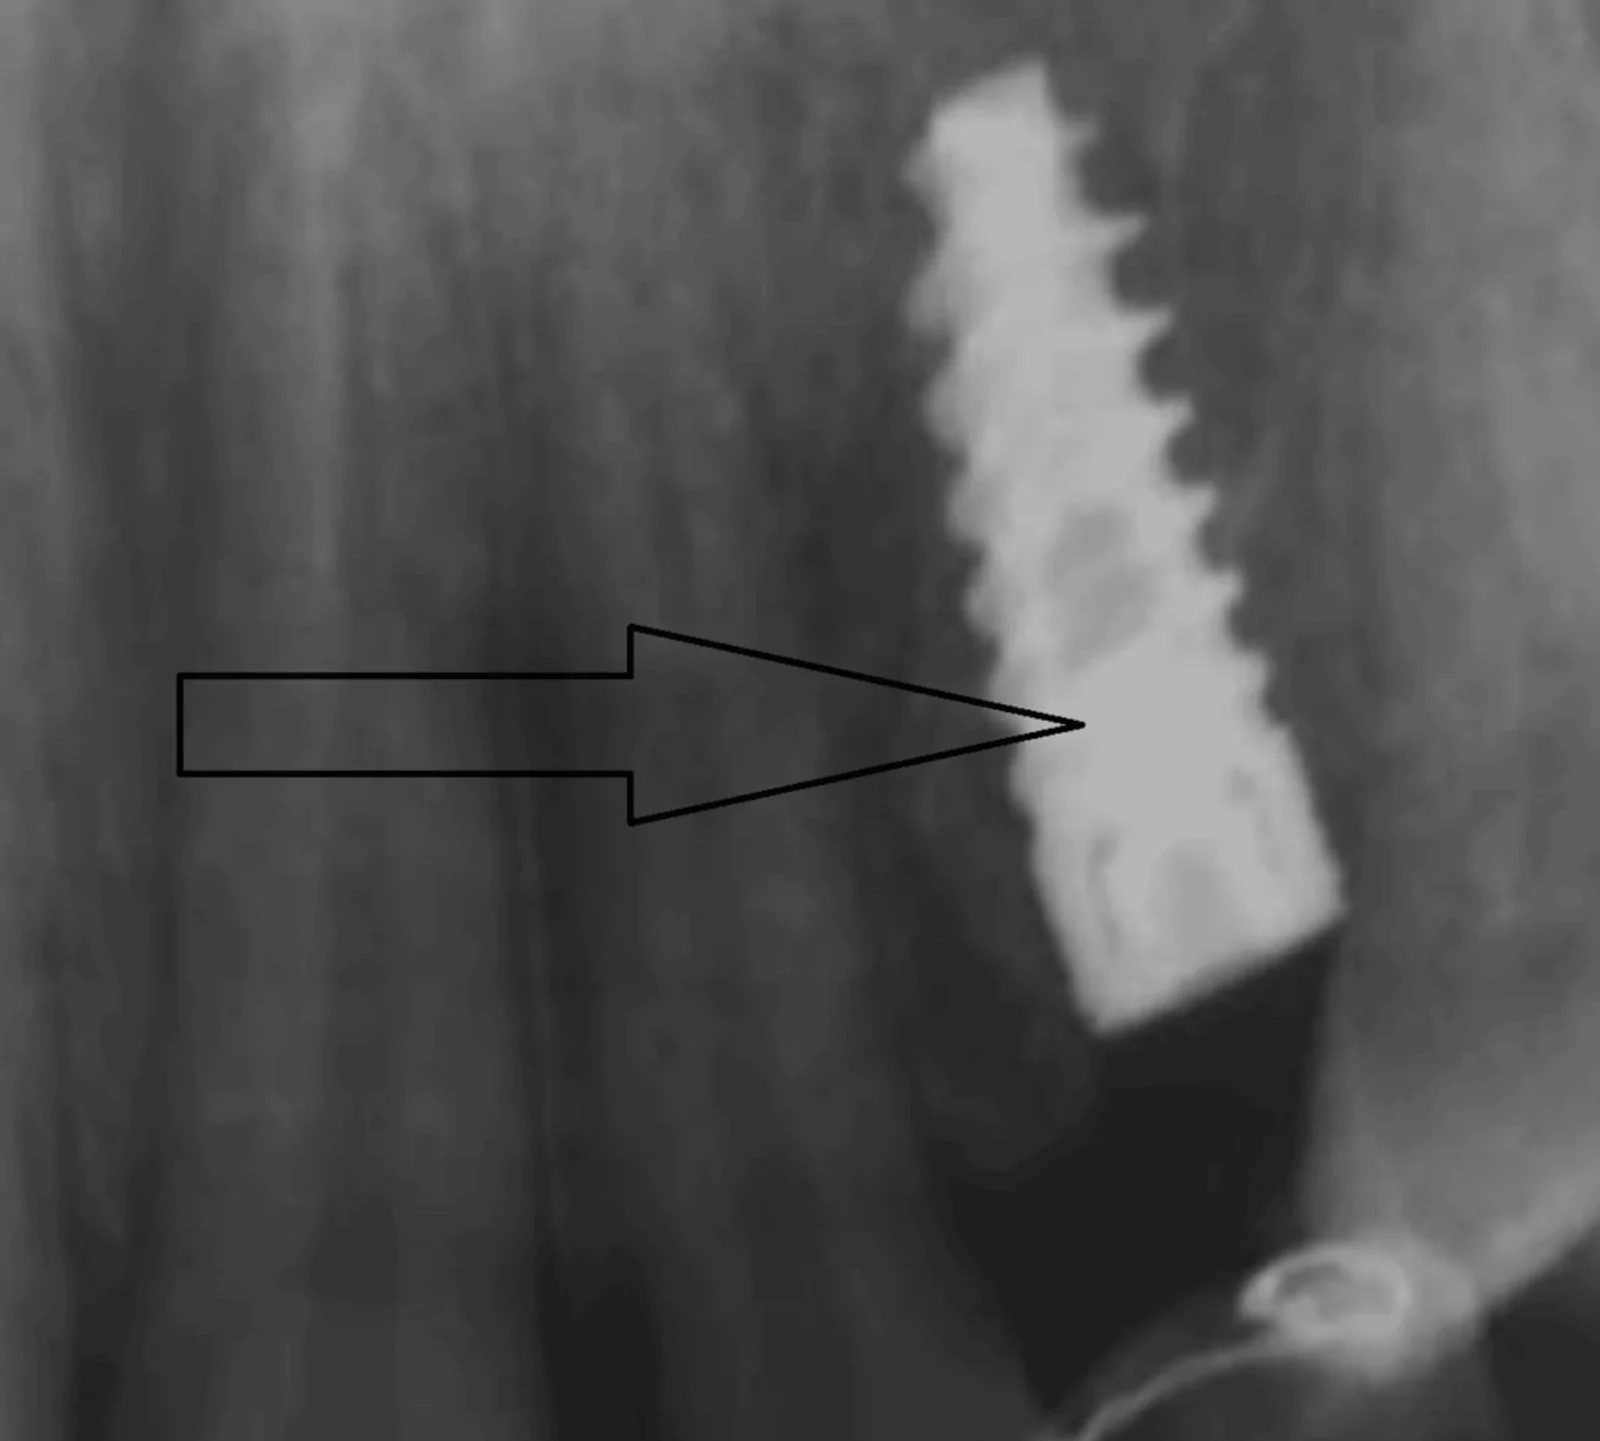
Postoperative Periapical Radiograph Following Implant Placement. Postoperative periapical radiograph demonstrating successful placement of the dental implant within the healed alveolar ridge after six months of ridge preservation. The black arrow indicates the implant positioned in the planned prosthetic location with satisfactory alignment and primary stability.
